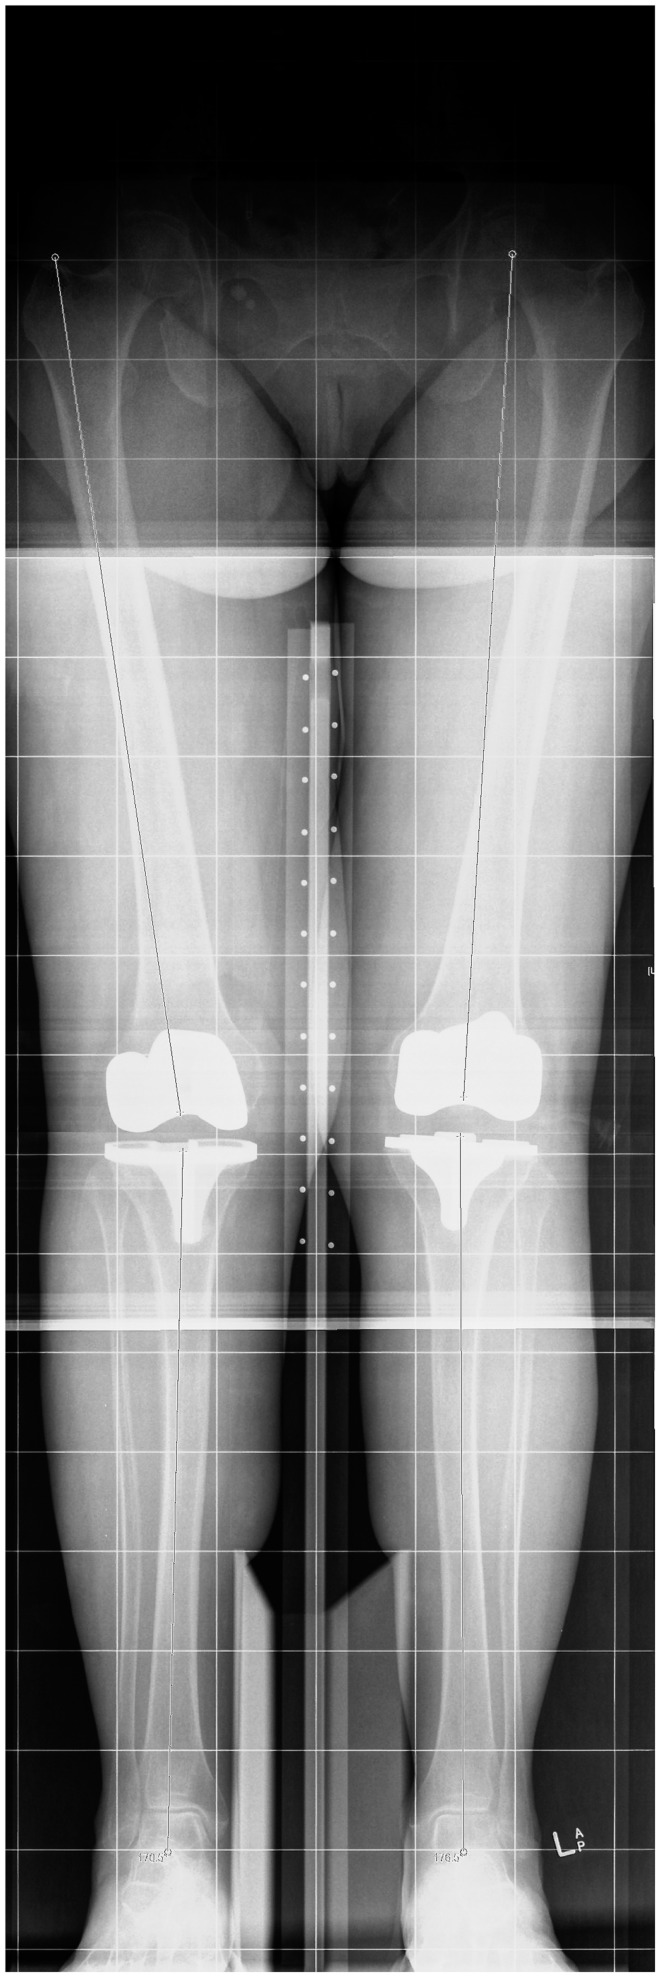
Measurement of mechanical and anatomical axes on the long-leg X-ray.
